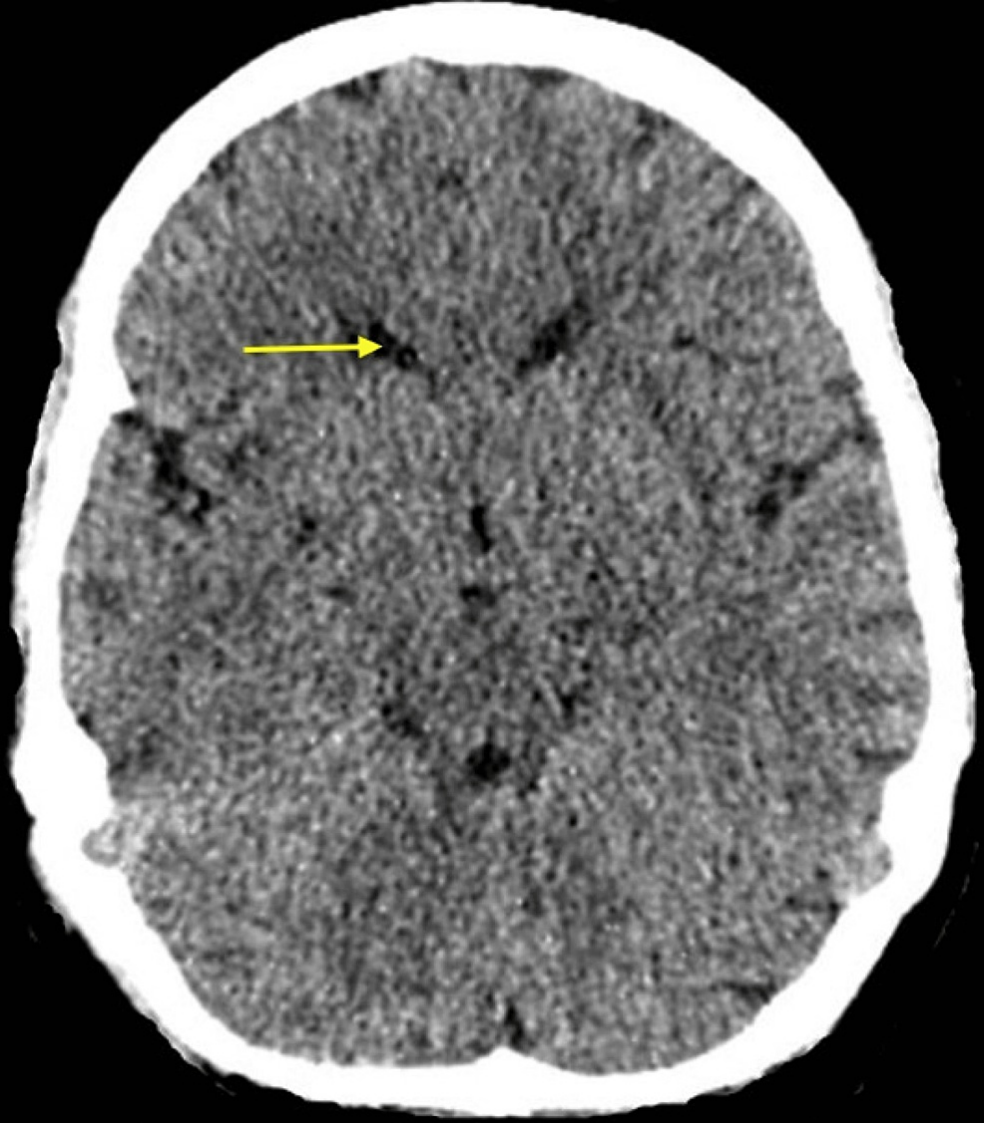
Computed tomography (CT) scan of the head Normal appearances of the brain parenchyma with preserved grey-white matter differentiation. No hyperdensity is identified on the venous sinuses to suggest underlying venous sinus thrombosis. Normal ventricular configuration (yellow arrow). No midline shift or hydrocephalus. No destructive skull lesions.
